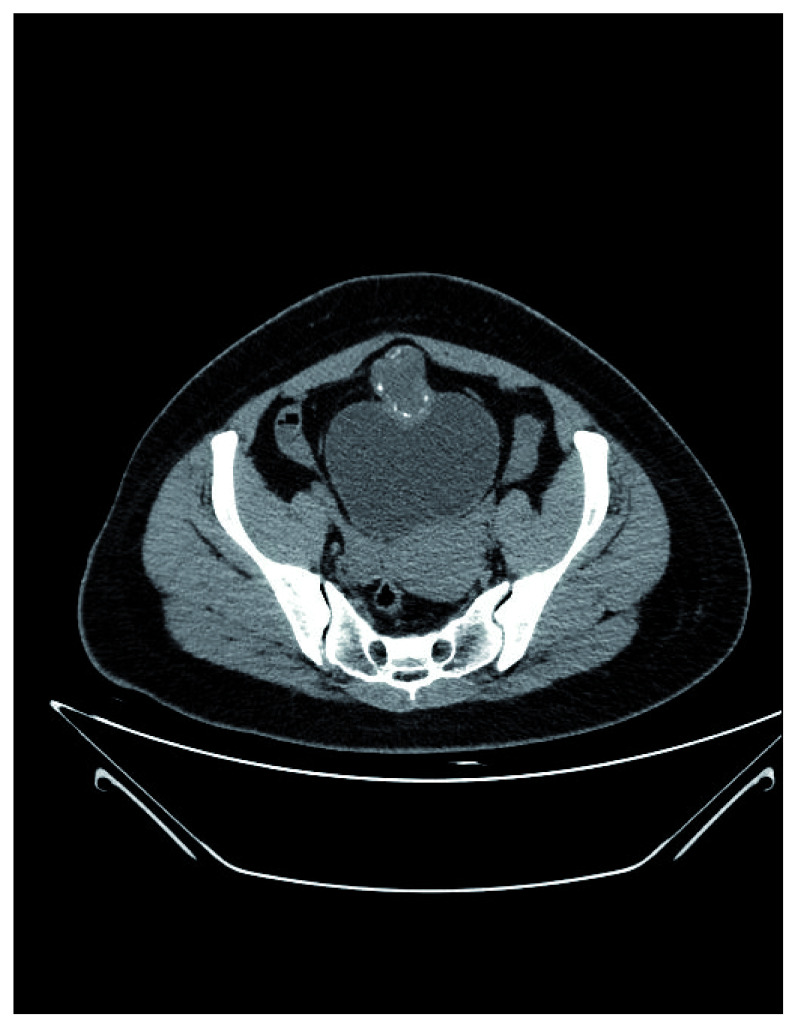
Lower abdominal CT scan showing superior and anterior bladder mass and cystic lesions with peripheral calcifications.
